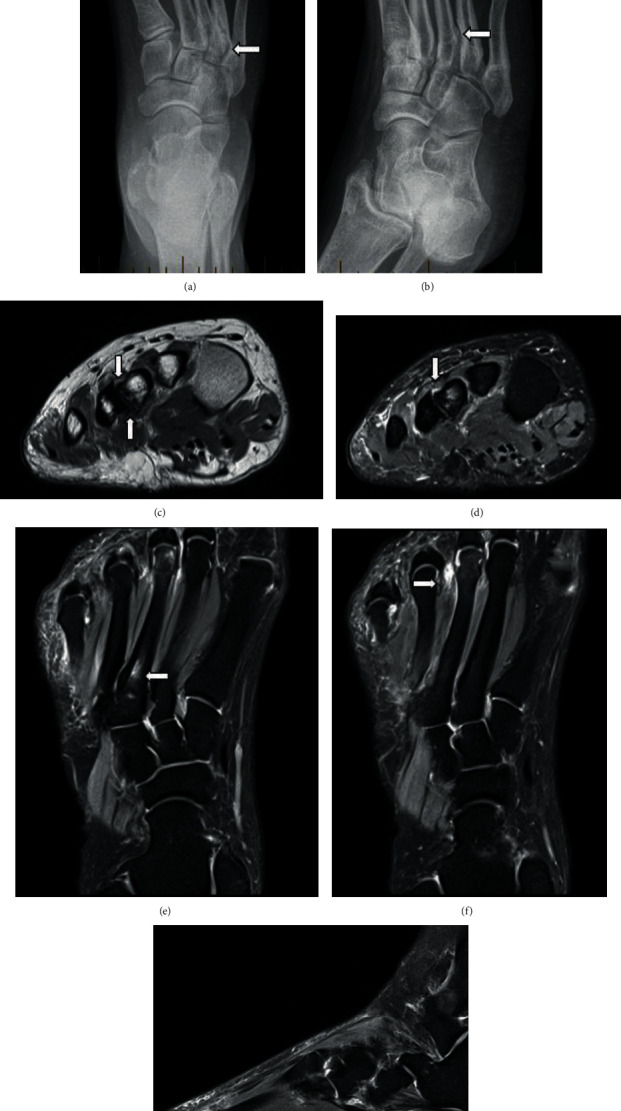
40-year-old female with dorsal forefoot pain following a change in activity. AP (a) and oblique (b) radiographs reveal bony prominence of the lateral border of the proximal third metatarsal shaft. Short axis PD (c) and PDFS (d) sequences reveal abnormal articulation between the base of the third and fourth metatarsals. Surrounding reactive bony hypertrophy and intramedullary marrow oedema signal. This is consistent with nonosseous intermetatarsal coalition. Coronal PDFS (e) sequence with nonosseous coalition between the base of the third and fourth metatarsals. Localized marrow oedema signal at the third metatarsal base. Coronal PDFS (f) sequence reveals a bursal-neuroma complex at the third intermetatarsal space corresponding to the location of the coalition. Sagittal PDFS sequences (g) with low-grade marrow oedema in the third metatarsal head. These are speculated to arise from the altered weight-bearing mechanics as a result of the coalition with increased load on the metatarsal head.
